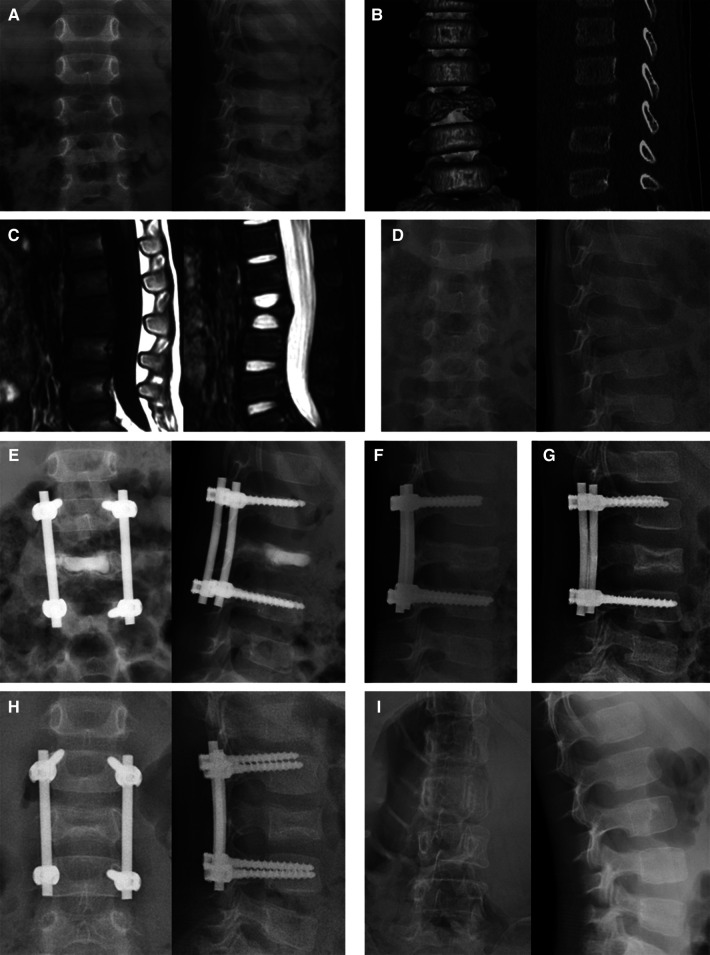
Series images of case 1, a 36-month-old boy with L3 vertebra plana. The patient took the plain x-ray (A), CT (B), and MRI (C) in the local hospital 2, 3, and 4 weeks after the symptom of back pain presented, respectively. These series images showed the aggravating damage of the L3 vertebra body. Two months after the initial symptom, the x-ray image (D) showed Grade IIA destruction of the L3 vertebral body. Immediately after the operation, vertebral height was partly restored (E). The calcium sulfate cement was completely absorbed in the 3-month follow-up (F). The vertebral height increased at the 6-month follow-up and osteosclerosis was observed (G). One year after the operation (H), an 89.2% recovery of vertebral height was observed and then the internal fixations were removed. Two years after the first operation (I), the vertebral body was well remodeled.
